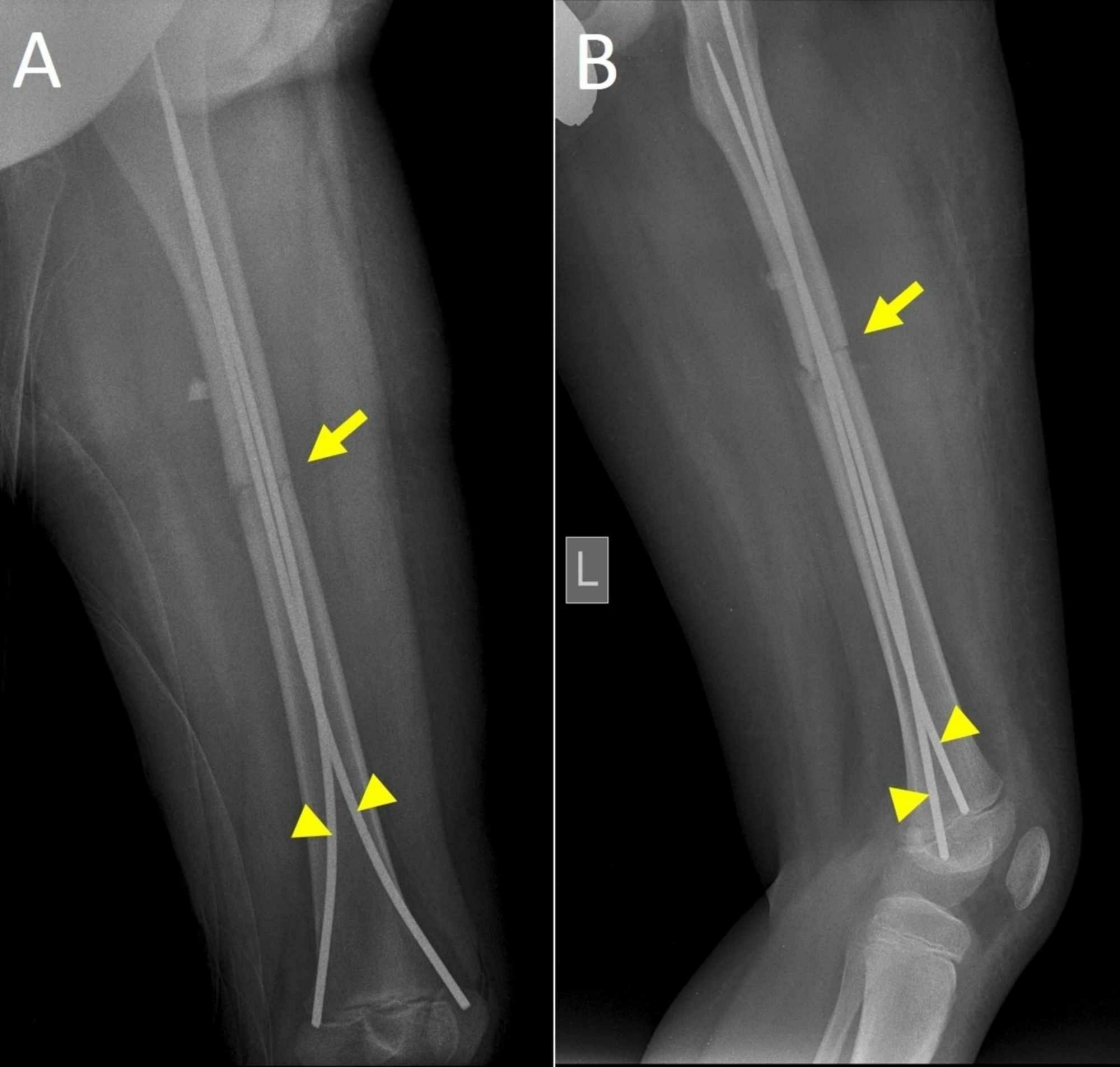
Postoperative X-ray after elastic stable intramedullary nailing (ESIN) of the left femur A. Anteroposterior view, B. Lateral view. Arrows indicate the fracture after reduction and osteosynthesis. Arrowheads indicate the titanium nails. Slight diastasis of the fracture fragments on both views is seen. Loss of femoral antecurvatum is evident on the lateral X-ray view.
